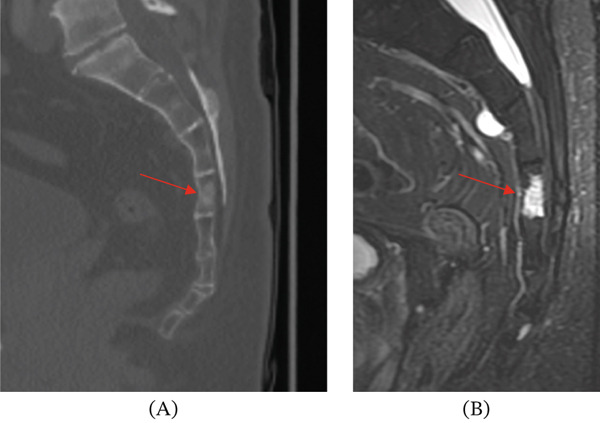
These images show the sacral lesions in our patient at S2–S3 and S4. (A) Panel A shows an intraosseous sclerotic lesion at S4 (red arrow) on sagittal CT. (B) Panel B shows the S4 lesion (red arrow) with a high signal on T2 STIR. These features at S4 are more typical of a BNCT.
